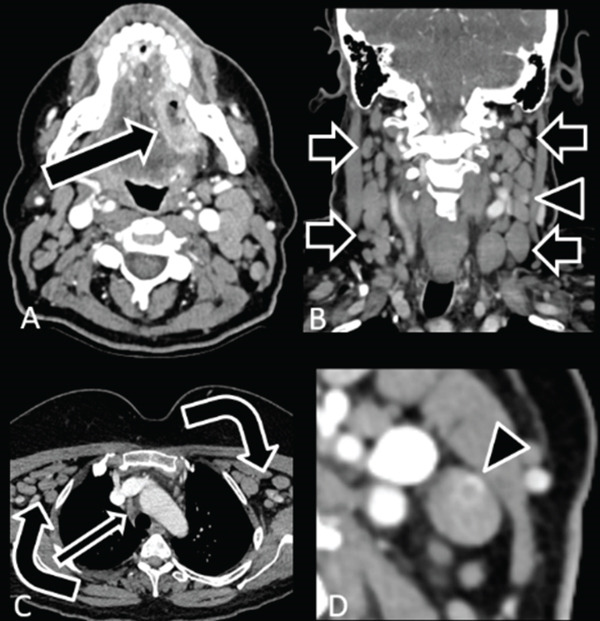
A 53‐year‐old female with a history of chronic lymphocytic leukemia (CLL) presented with biopsy‐proven left oral tongue squamous cell carcinoma (SCC). (A) Axial contrast‐enhanced soft tissue neck CT shows an enhancing ulcerated mass in the left oral tongue (arrow). (B) Coronal image demonstrates diffuse bilateral nonnecrotic lymphadenopathy in the neck (short arrows), which is typical for leukemia/lymphoma and not typical for metastatic SCC. (C) Axial image at the level of the upper chest shows nonnecrotic bilateral axillary lymphadenopathy (curved arrows) and mediastinal lymphadenopathy (thin arrow), typical for leukemia/lymphoma. In this case, node size cannot be reliably used to assess for metastatic SCC. Careful inspection, however, reveals a small internal ring of enhancement in a left Level IIB node: (B) arrowhead in coronal image and (D) arrowhead in axial image, which is less typical for leukemia/lymphoma.
